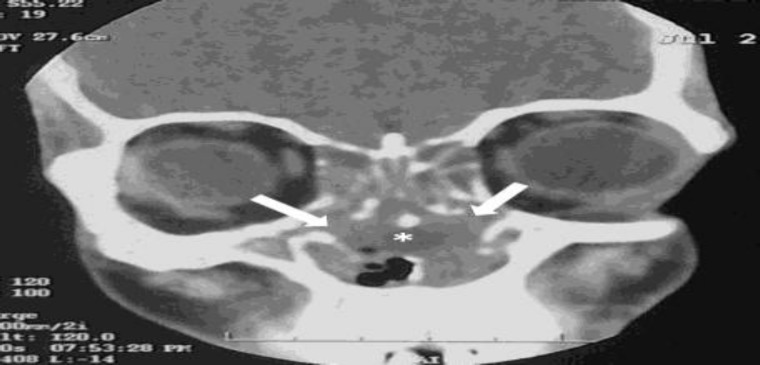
Coronal CT image shows destruction of nasal septum (asterisk) with mass filling the nasal cavity (arrows
